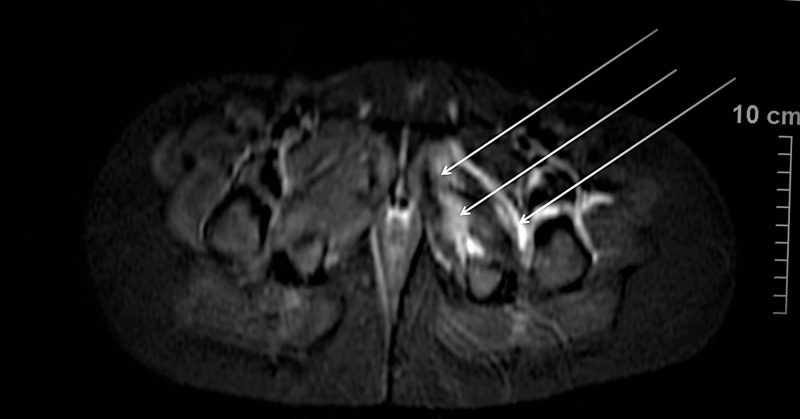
Magnetic resonance imaging (MRI) scan of a 10-year-old boy. Arrows point to the fluid concentration within or around muscles.
